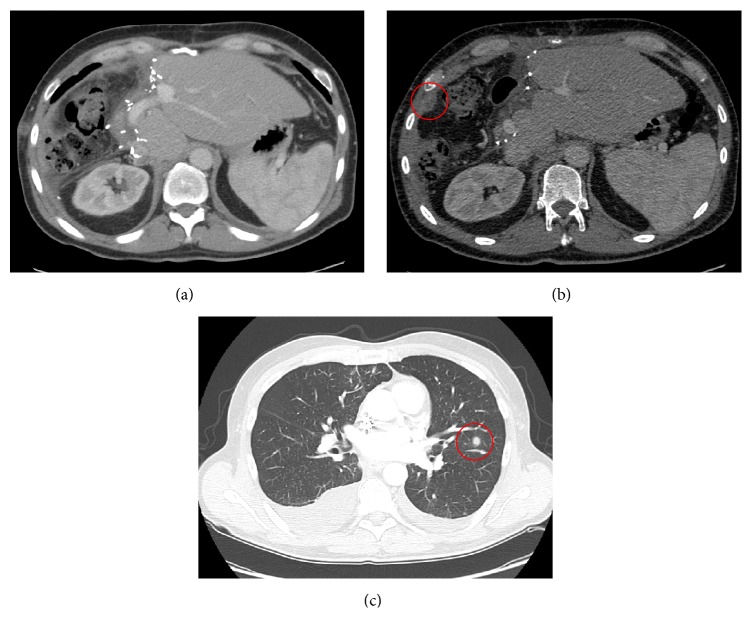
Follow-up CT-scan. Follow-up CT-scan approximately 2.5 months after liver resection showing the further volume increase of the liver remnant (a). Furthermore, examples of the novel extrahepatic tumor manifestations in terms of peritoneal carcinosis (b) as well as lung metastases (c) are shown (marked by red circles, resp.).
